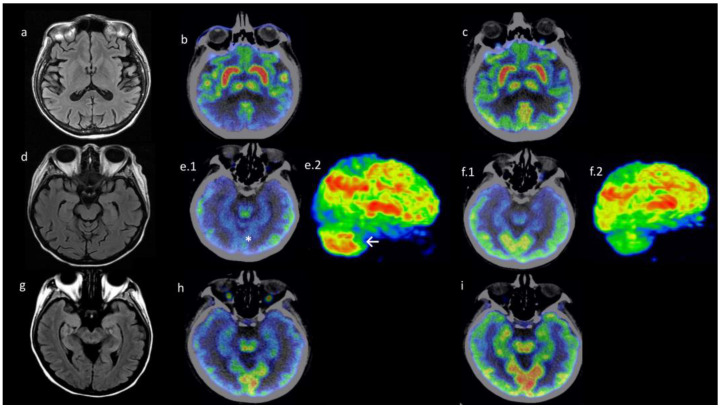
Immunological findings in MRI and PET/CT images before and after treatment. Left column: Magnetic resonance images. Central column: Pre-therapy PET/CT images. Right column: Post-therapy images. Upper row: Case #1. (a) FLAIR MRI does not show any abnormality. (b) For semiquantitative analysis with CortexID software, we found a Z-score of −7.7 and −5.4 for occipital and frontal lobes, respectively. However, the mesial temporal region and cerebellum showed Z-scores of +7.9 and +2. (c). After treatment, all Z-scores demonstrated better values (occipital −3.1, frontal −2.4, mesial temporal +2.8, cerebellum −0.06). Middle row: Case #2. (d). FLAIR MRI did not show any abnormal findings. Panels (e.2) and (f.2): FDG maximum intensity projection. Panels (e.1) and (e.2). Brain FDG PET/CT. Cortex ID showed a Z-score of −7.25 for the frontal lobe and −2.7 for the occipital. Higher positive Z-score were found in mesial temporal +2.47 and cerebellum +4.66 (*), Negative Z-score values showed improvement after therapy (frontal +2.5, occipital −1.68). Temporal mesial turned negative with a Z-score of −2.39, and cerebellum was normal (−0.4) (←), (Panels (f.1) and (f.2)). Lower row: Case #3. (g). FLAIR MRI with increased signal intensity in both mesial temporal lobes. (h) Cortex ID showed a Z-score of −7.58 for the frontal lobe and −2.17 for the occipital. Higher positive Z-score were found in the mesial temporal +5.84 and cerebellum +5.6. (i) FDG PET post-therapy showed normal metabolism.
